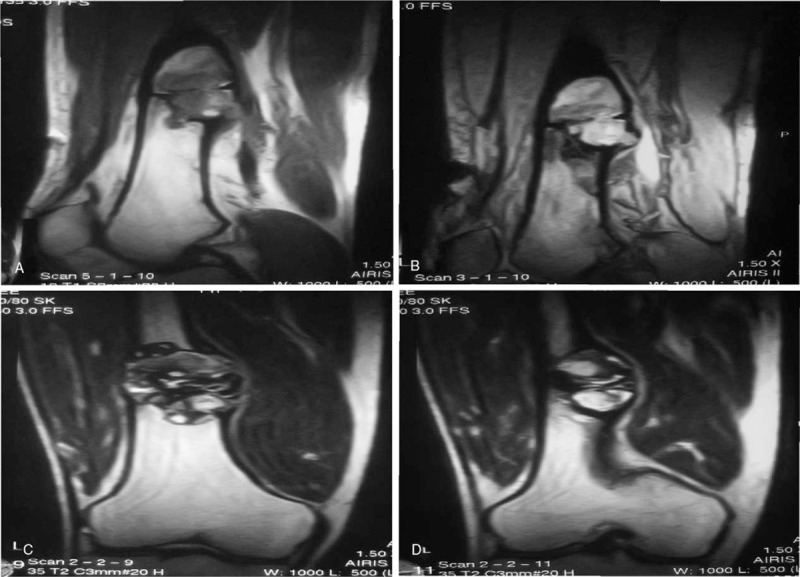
Magnetic resonance imaging of the lesion demonstrated heterogeneous signal intensity, with hypointensity and hyperintensity signal on T1 (A), T2 (B), and T2-weighted images (C&D).
